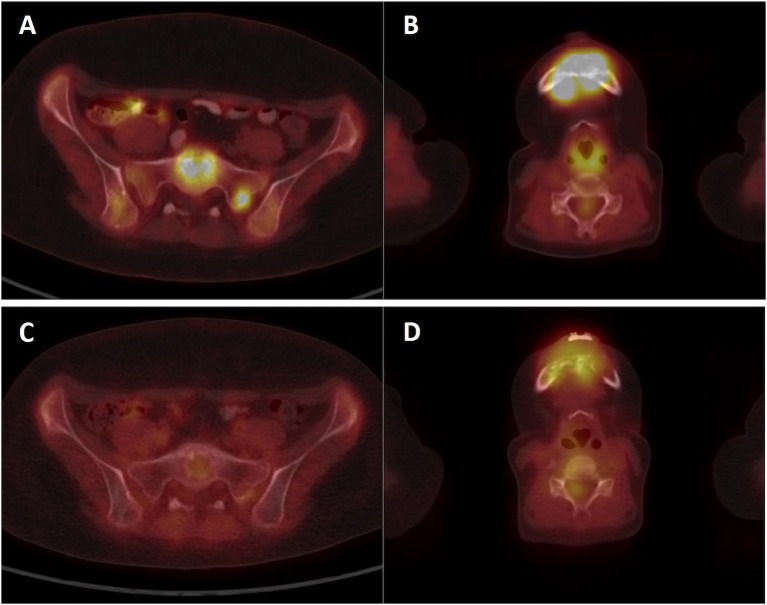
PET/CT imaging of patient KDO-0101. (A,B) Examination of patient at second relapse in July 2017 showed ^18^F-FDG-positive osteolytic lesions in the skeleton (A) sacrum, sacral base with a target-to-liver ratio of 2.74 and sacral left lateral mass with a target-to-liver ratio of 2.39 (B) mandible with a target-to-liver ratio of 4.88. (C,D) Control ^18^FDG-PET/CT examination in January 2018 showed a decrease or complete diminishment of ^18^FDG accumulation (C) sacrum, sacral base with a target-to-liver ratio of 0.69, and sacral lateral mass with a target-to-liver ratio of 0.66 (D) mandible with a target-to-liver ratio of 1.47.
